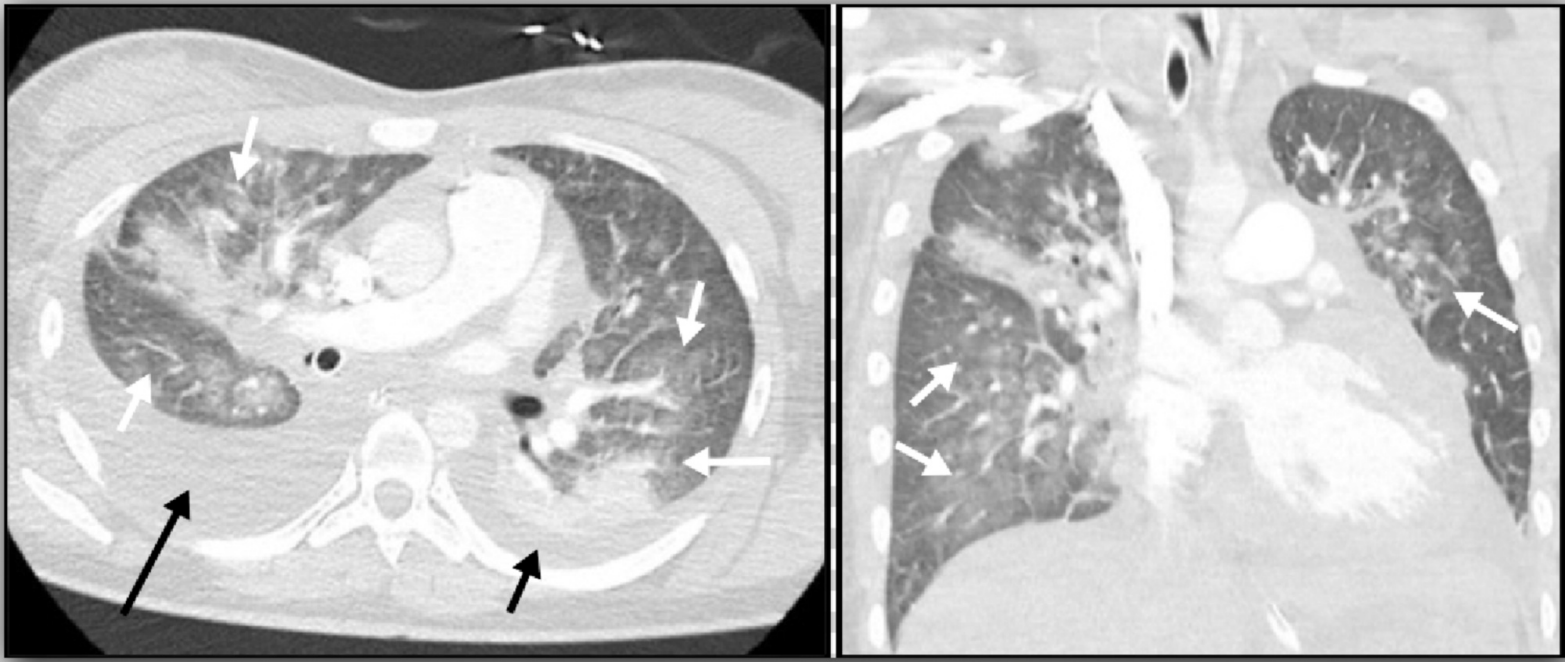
Chest CT scan with intravenous contrast demonstrates evidence of pulmonary edema (white arrows) with small left (short black arrow) and moderate right-sided pleural effusion (long black arrow).
